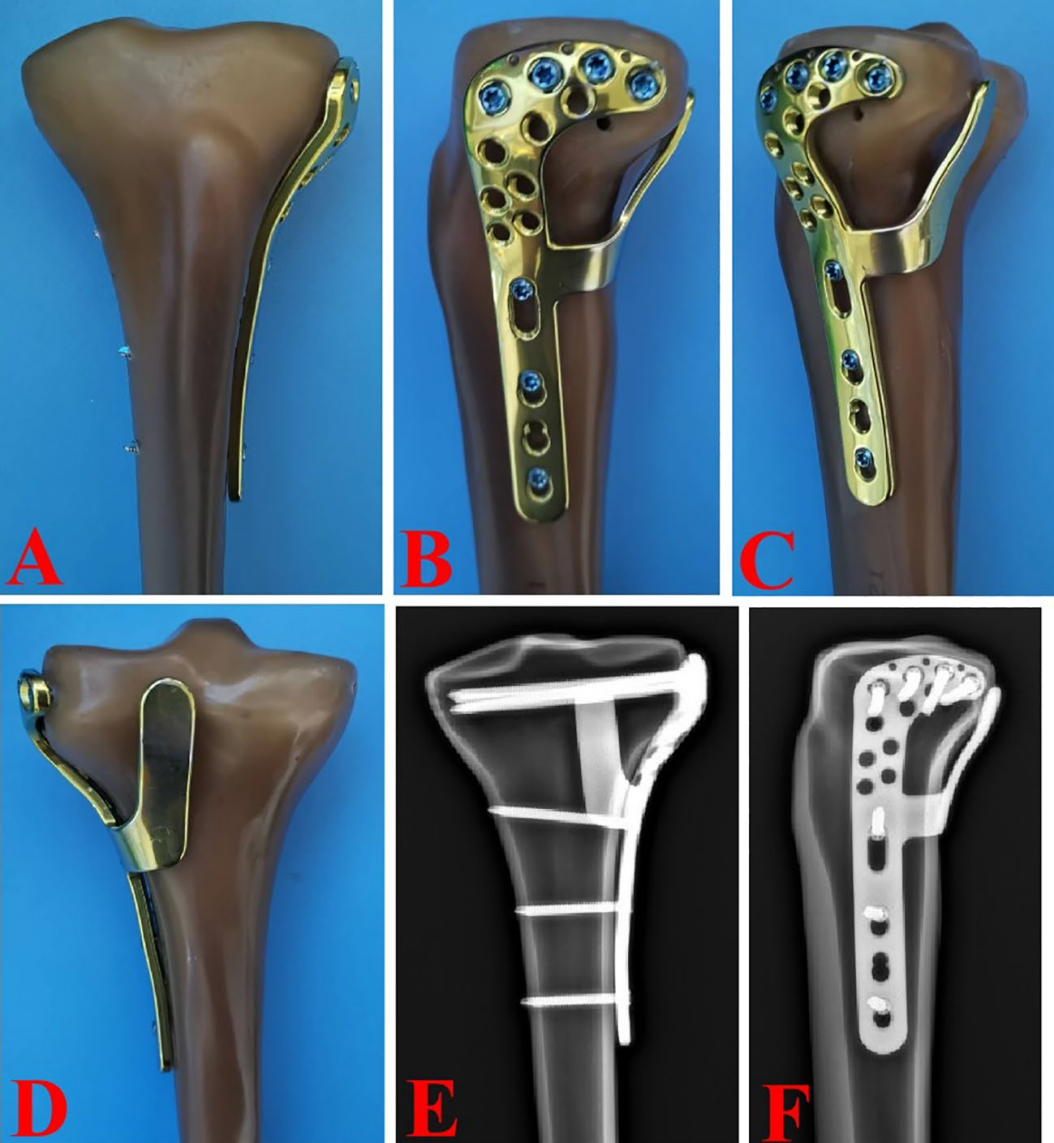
Mock-ups of the novel plate in synthetic bones. (A–D) Anterior, lateral, posterolateral and posterior views of the novel plate. (E,F) Anteroposterior and lateral view of the X-ray images.
